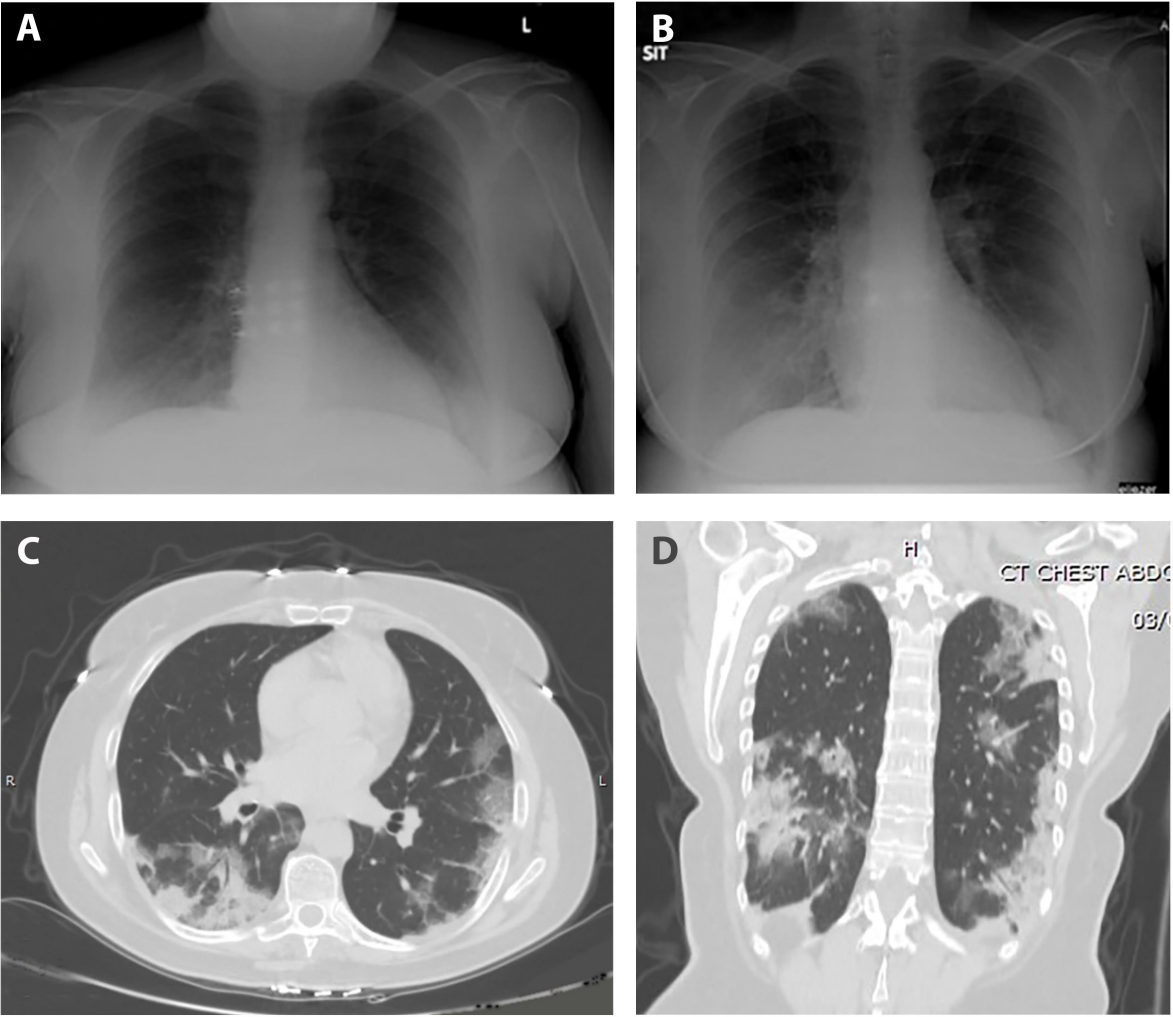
Anteroposterior chest radiograph; (A) normal on admission, (B) on day 8 of hospitalization with bilateral basal infiltrates. Axial (C) and coronal (D) sections CT scan; bilateral multifocal peripheral pulmonary ground glass opacities and consolidations typical for COVID19 infection.
